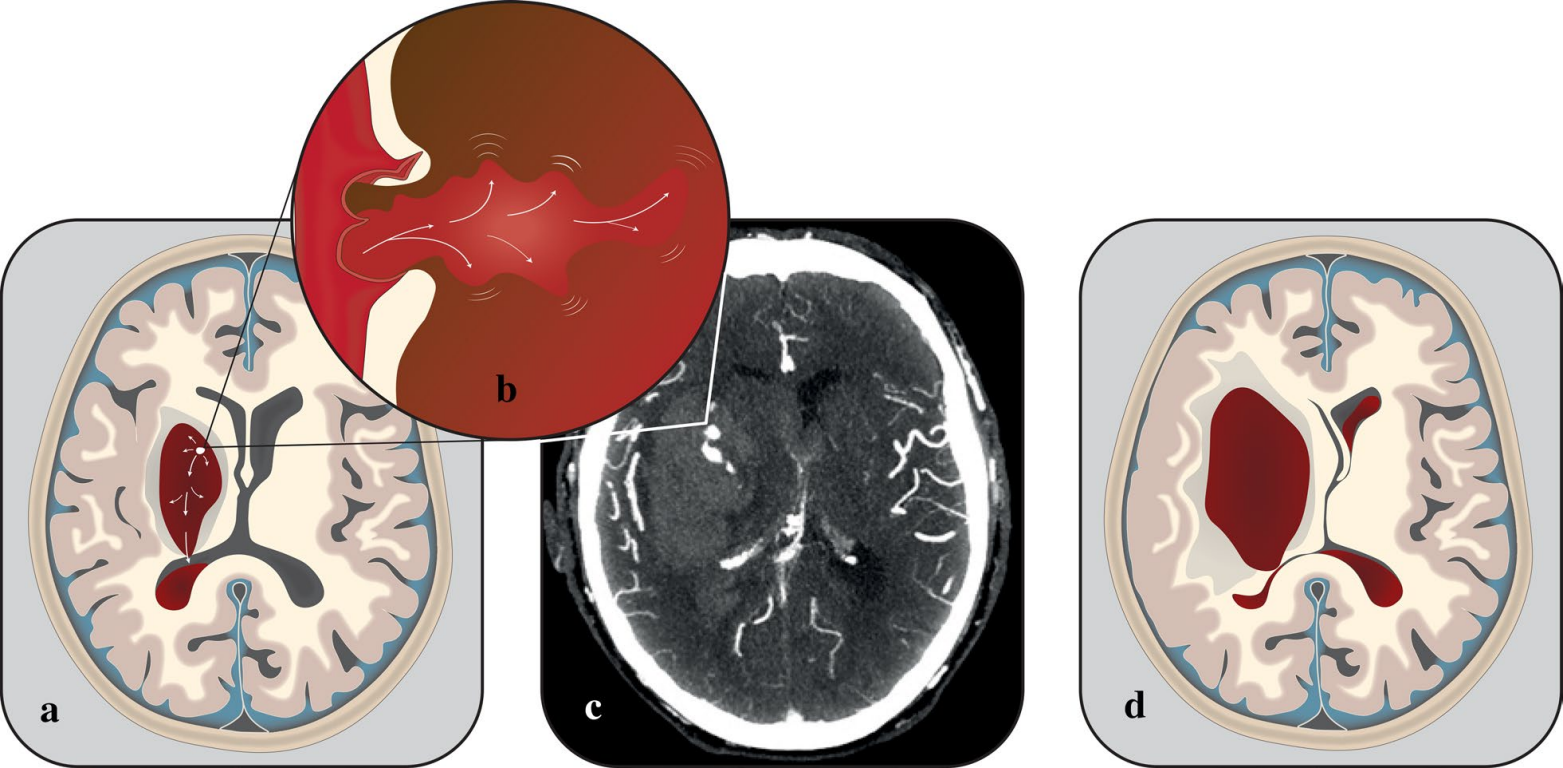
The spot sign is generally understood to be a radiological visualisation of an actively bleeding ruptured blood vessel (b) causing intraparenchymal haematoma expansion and by extension expansion of the intraventricular haematoma (a). The actively bleeding ruptured vessel is visualised on CT-angiography as the spot sign. c Depicts an authentic CT-angiography image of an acute intracerebral haemorrhage patient. A relatively large spot sign can be observed within the haematoma. Significantly enlarged intraparenchymal and intraventricular haematomas can often be seen on follow-up imaging (d)
